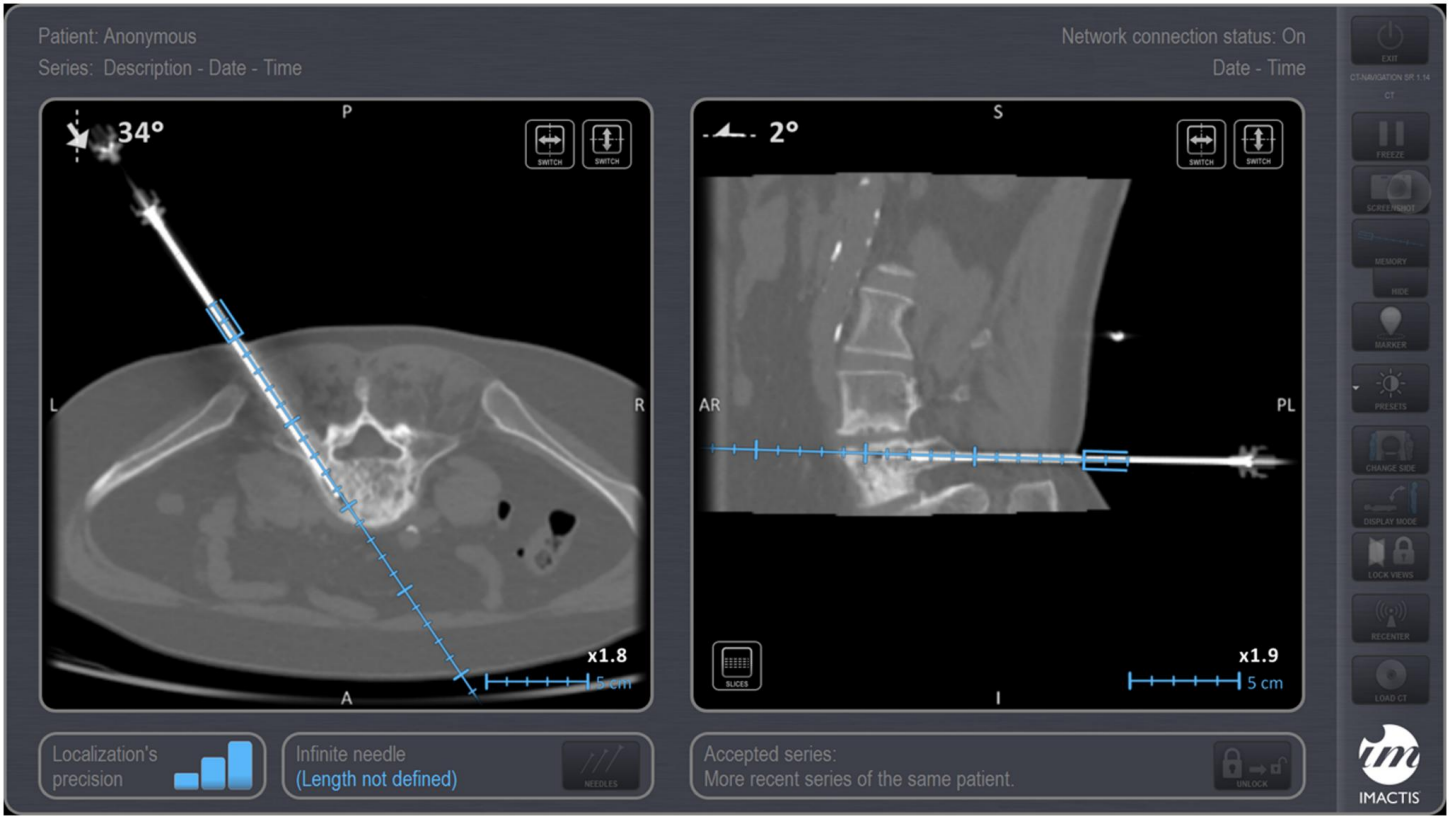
A 51 y-o female breast cancer patient with a mixed L5 lesion underwent percutaneous biopsy under CT guidance using an electromagnetic navigation system. Notice the accuracy and lack of Euclidian error between the planned (blue line) approach as selected in the planning screenshot and the biopsy needle as placed in the final control screenshot.
